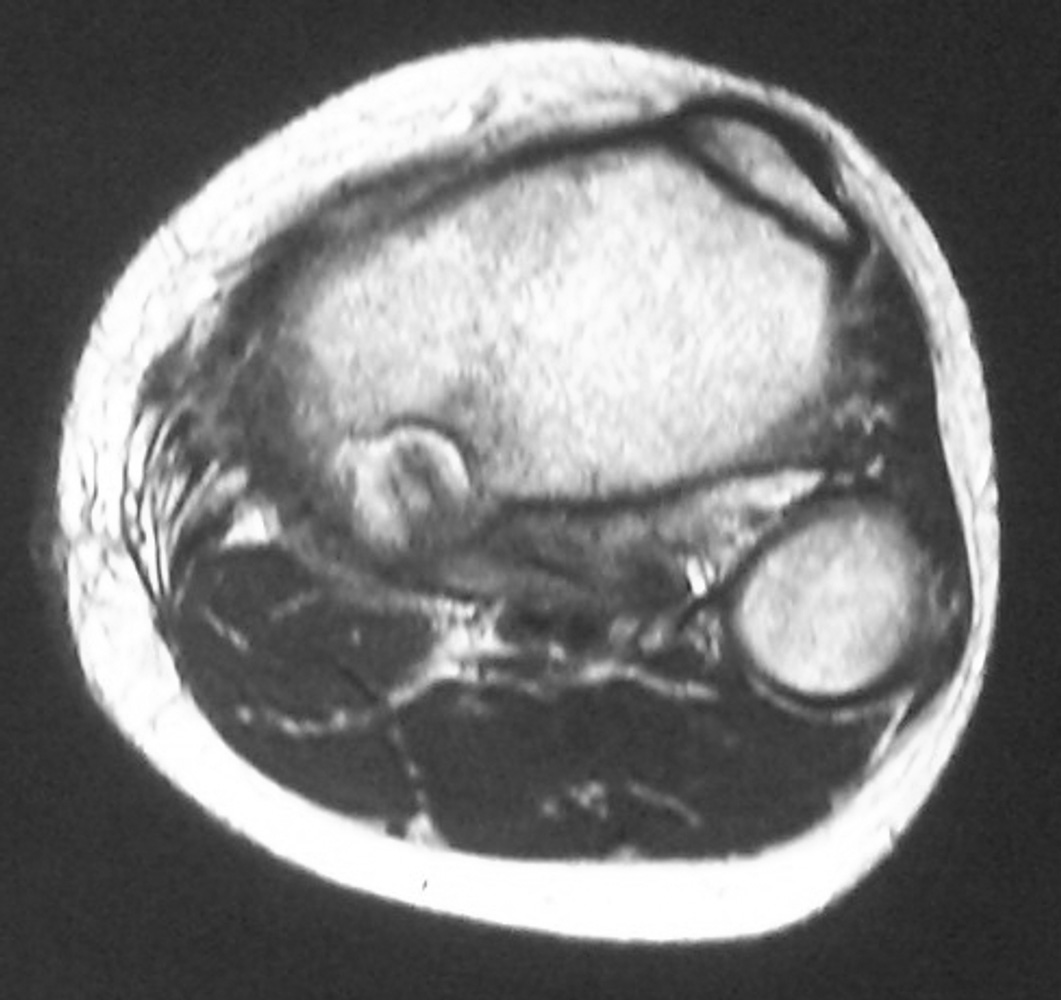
MR imaging 2 months after surgery. Soft tissue edema reduced markedly.
